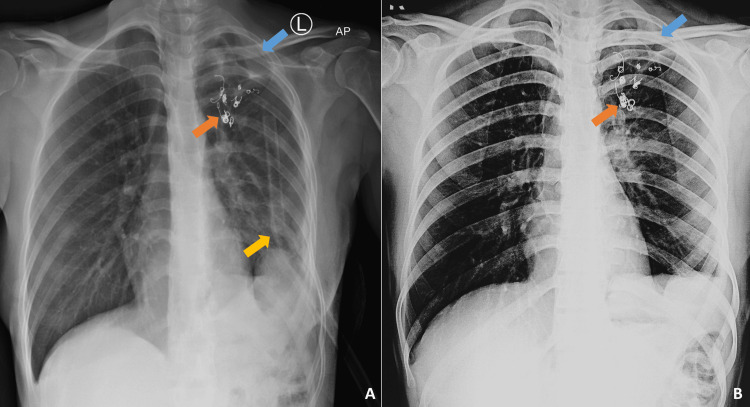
Post-embolization and follow-up imaging (A) Chest radiograph obtained two days after coil embolization and glue placement, showing near-complete expansion of the left lung with a small residual left pneumothorax (blue arrow). One chest tube has been removed, with the remaining tube in situ (yellow arrow). (B) Follow-up chest radiograph obtained 14 days after discharge demonstrating stable findings with near-complete left lung expansion and a small residual pneumothorax (blue arrow). Radio-opaque vascular embolization coils are visible in both panels (orange arrows).
